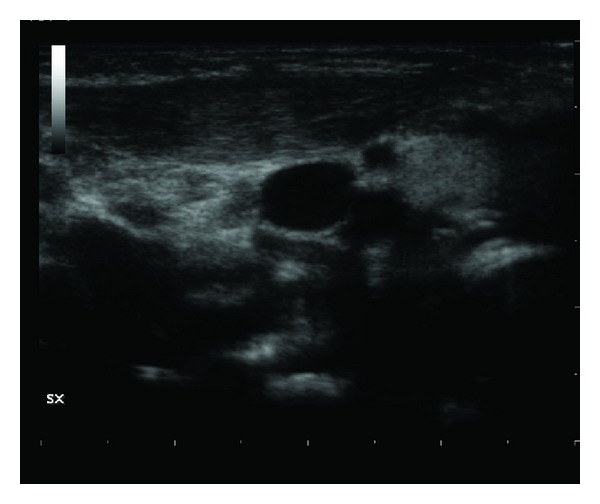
The ultrasound scan made after a month shows a marked improvement of the sternocleidomastoid muscle, with only an increase of myofiber in the third middle of the ventral belly.
